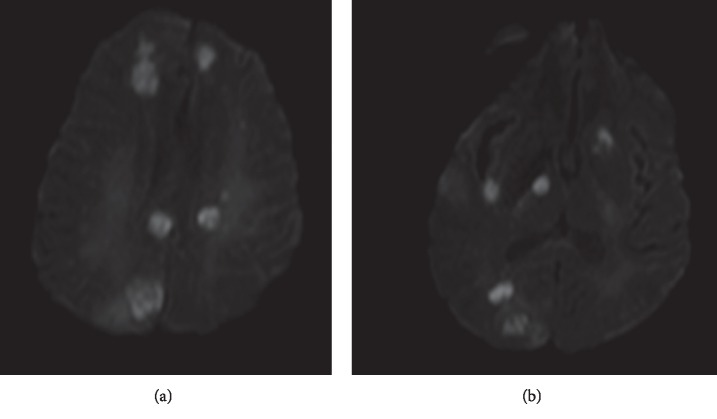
Image of MRI brain showing multifocal areas of diffusion restriction suggestive of vasculitis versus acute infarcts.
